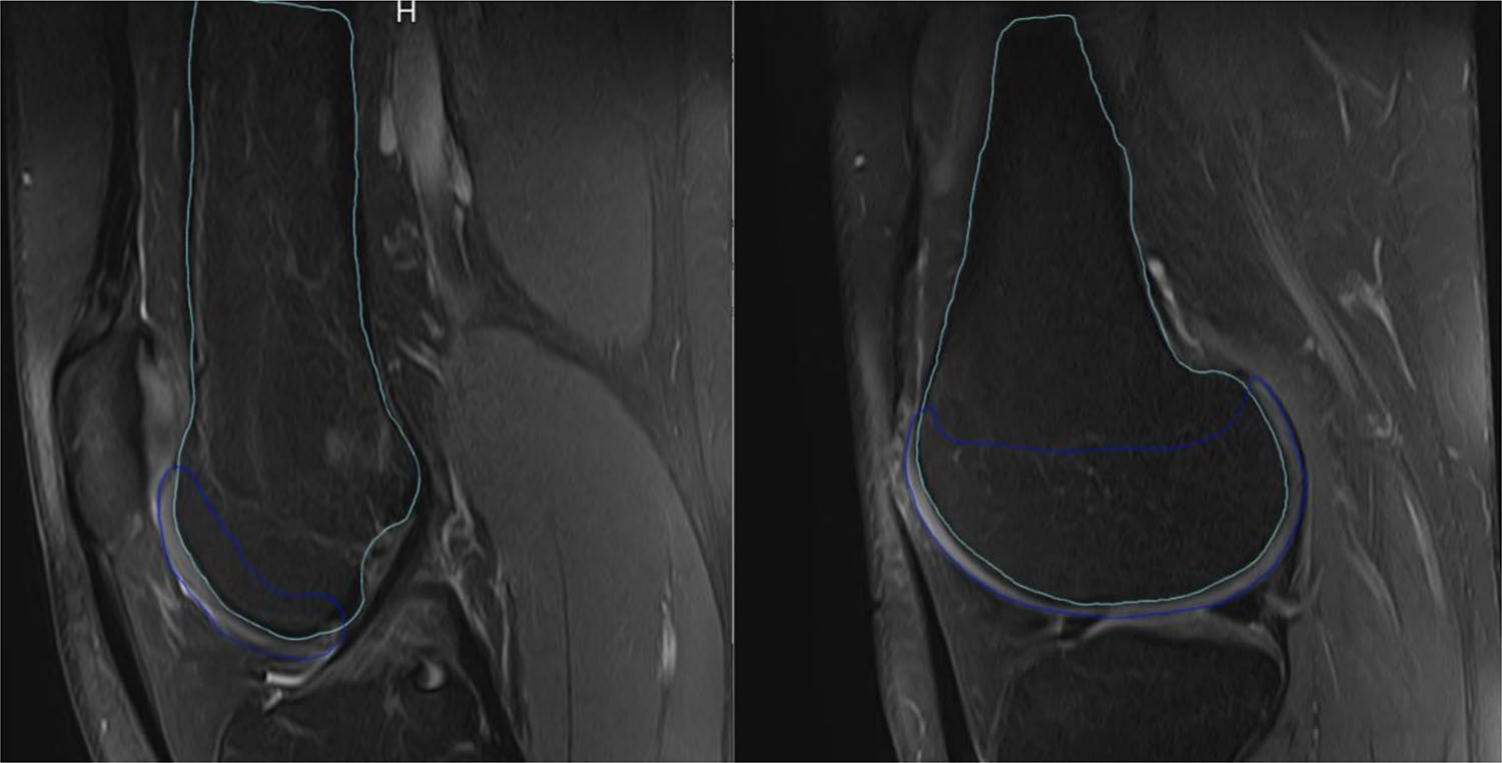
Segmentation of cartilage (blue) and bone (green) structures of the distal femur in T2 weighted sagittal MRI slices. *Blue line: segmentation of femoral cartilage in sagittal MRI slices for the creation of a 3D model; Green line: segmentation of femoral bone in sagittal MRI slices for the creation of a 3D model
